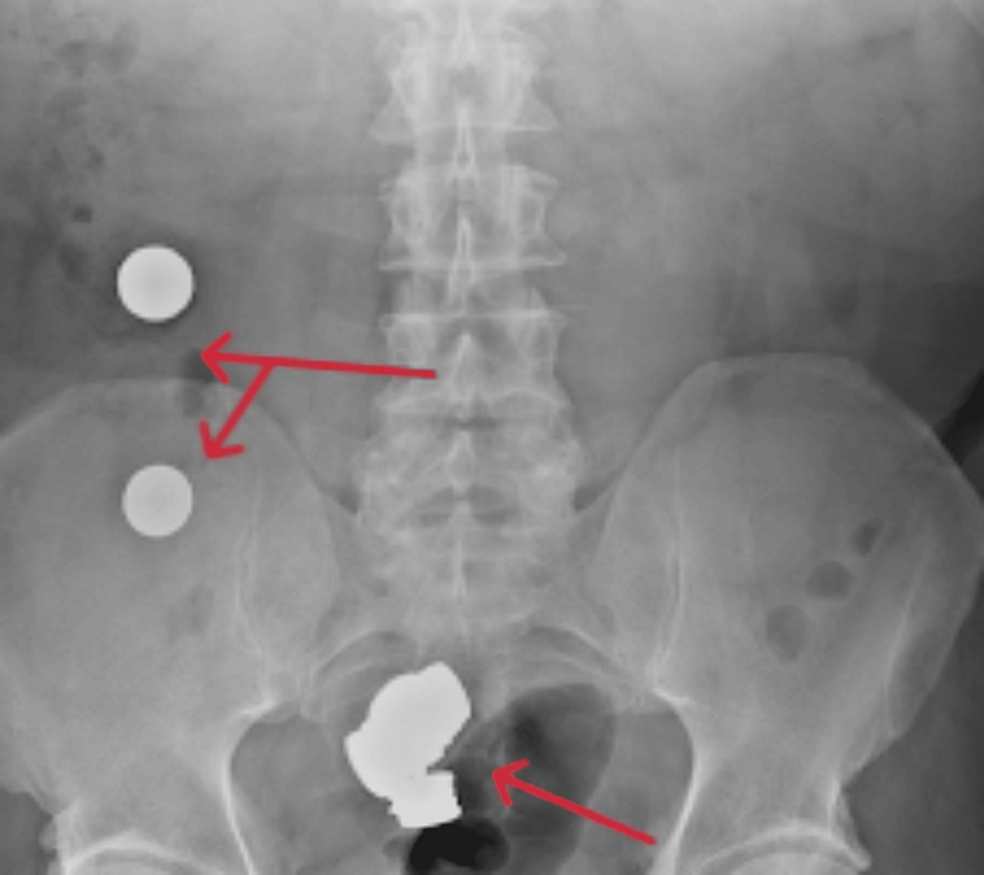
X-ray revealing multiple foreign bodies in the small bowel and cecum (arrows)
